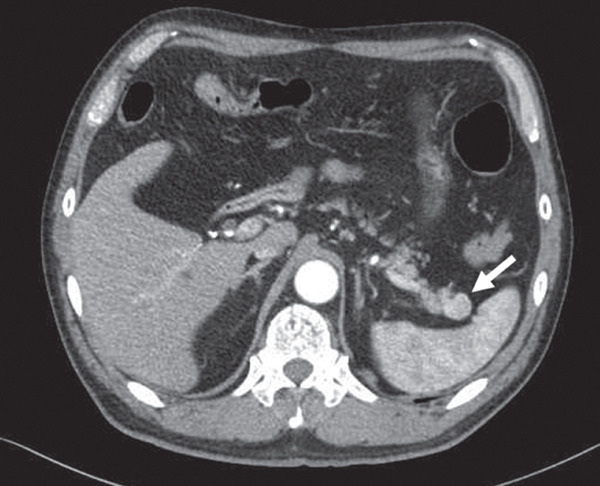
Computed tomography of insulinoma. Arterial‐phase pancreatic computed tomography shows a homogeneously enhancing mass, 20 mm in diameter (arrow), in the tail of the pancreas. On the portal venous phase, there was persistent enhancement as compared with the pancreatic parenchyma (not shown).
